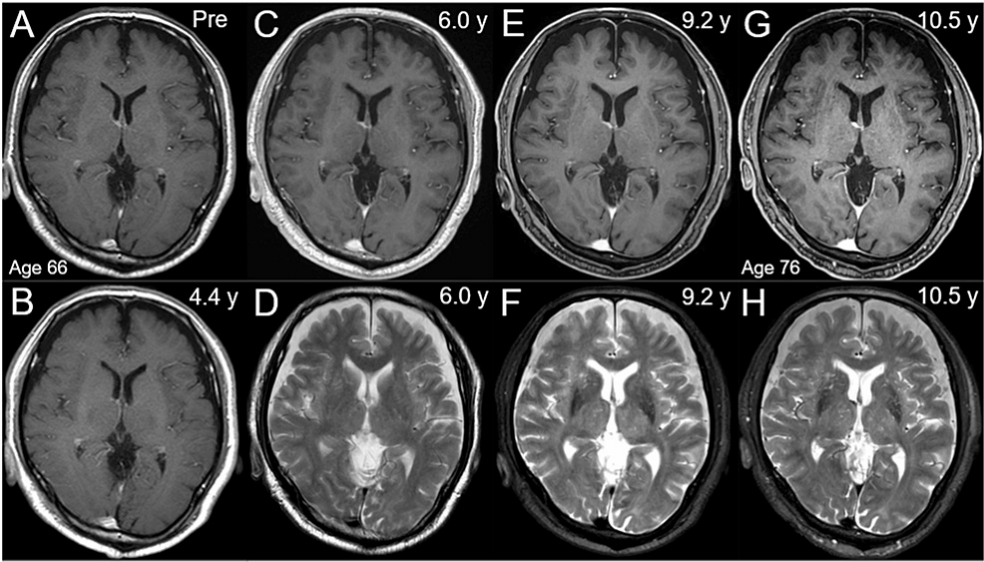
Morphological changes of the brain on the MRI before and after the initiation of anti-cancer treatment The images show axial CE-T1-WIs (A-C, E, G) and axial T2-WIs (D, F, H); before the first-line chemotherapy (Pre, at age 66) (A); at 4.4 years (y) after the first-line chemotherapy (at the time of detecting the tiny BM) (B); at 6.0 years (before the initial SRS) (C, D); at 9.2 years (before re-SRS) (E, F); and at 10.5 years (before initiating WBRT, at age 76) (G, H) (A-H) These images are shown at the same magnification and coordinates under co-registration and fusions. (A, B) T2-WIs were not acquired until six years after initiating chemotherapy. (A-H) Over the 10.5 years, the parenchymal morphology and white matter signal changes were minimal, and there was no obvious increase in bilateral subdural fluid collections MRI: magnetic resonance imaging; CE: contrast-enhanced; WI: weighted images; BM: brain metastasis; SRS: stereotactic radiosurgery; WBRT: whole brain radiotherapy
